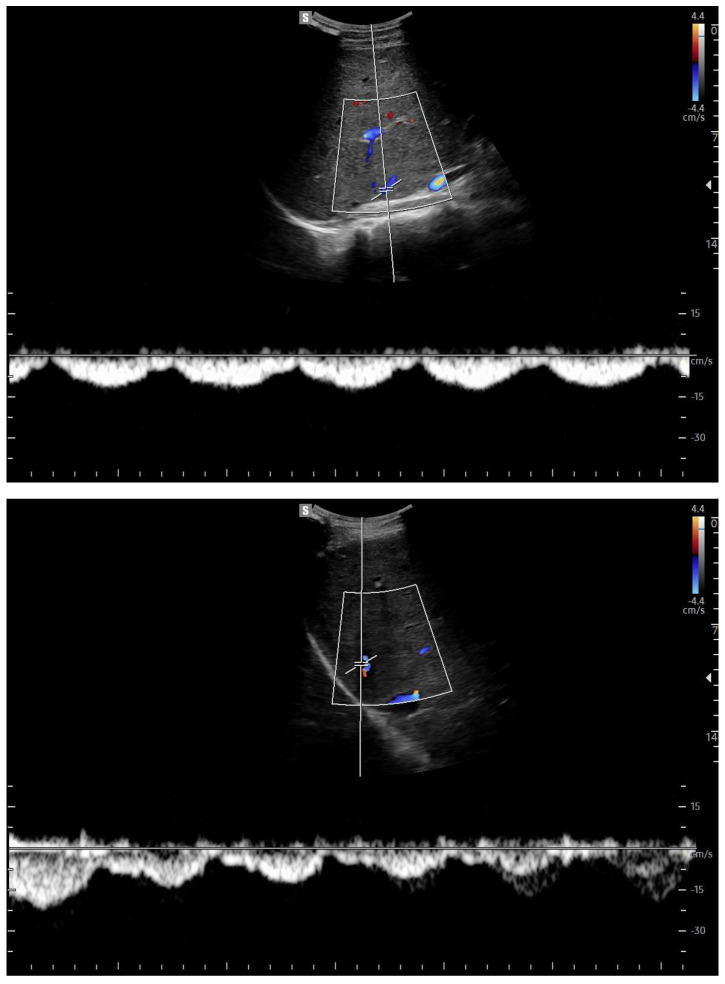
Doppler Ultrasound of the middle hepatic vein showing the loss of the normal three-phase pattern due to the absence of atrial contraction.
